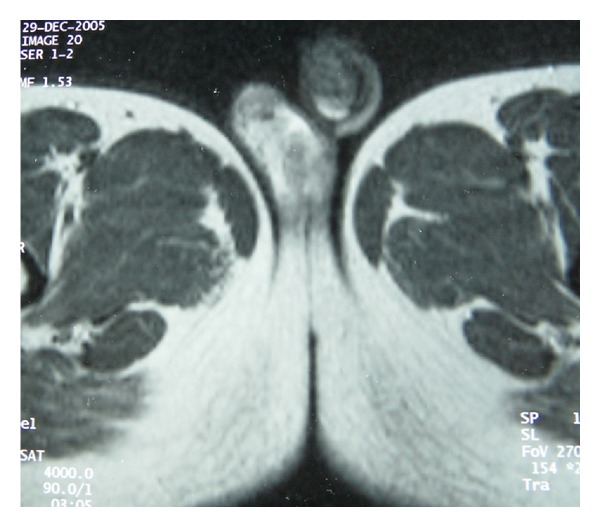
Testicular T2-weighted images showing bilateral hyperintense lesions with homogeneous enhancement.
